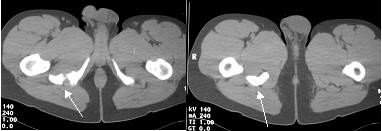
Pre-operative computed tomography images of the pelvis. The arrows point to the heterotopic bony masses.
